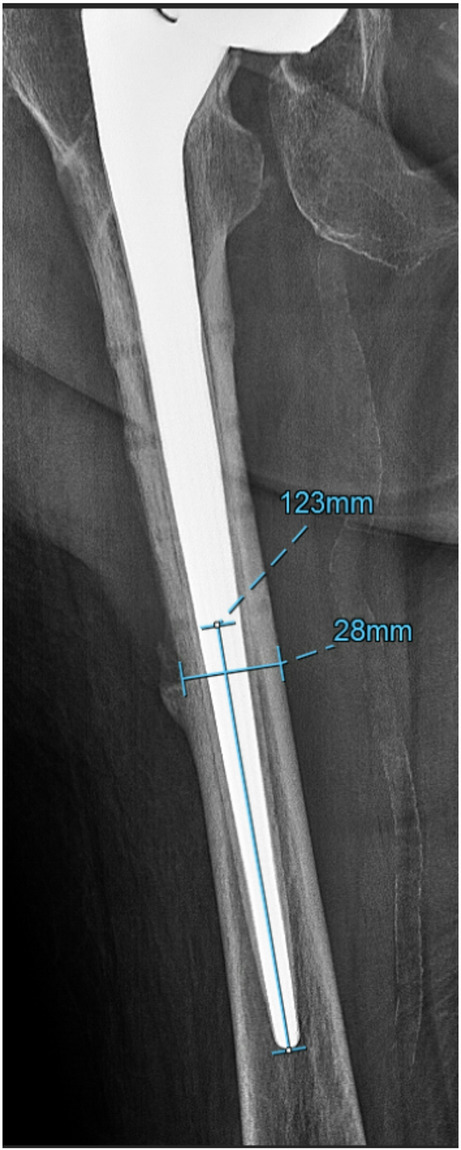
Representative radiographs showing measurement of SSD (stem to screw distance divided by diaphyseal width) measurement. Vertical measurement represents the distance of femoral stem that bypasses the most distal screw hole proximal to the femoral isthmus (SS). Horizontal measurement represents diaphyseal width
